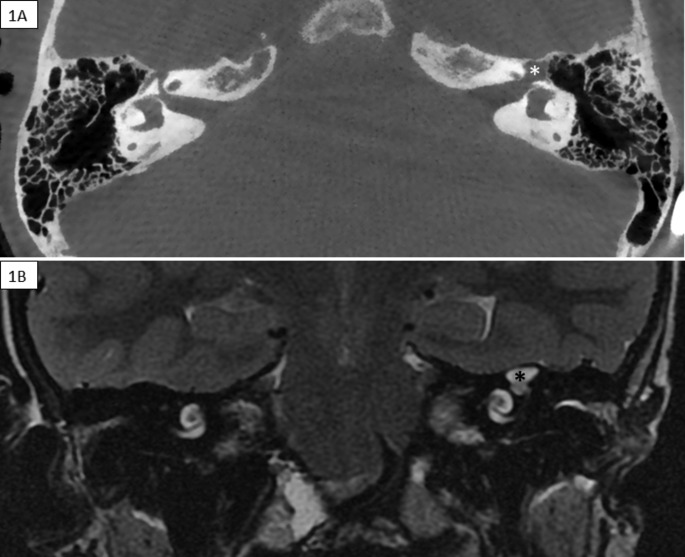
A (Cone Beam CT) and B (MRI, T2 sequence): Fluid ectasia in the left geniculate ganglion (*) in continuity with the tympanic and labyrinthine segments of the facial nerve. There are no bony defects communicating the ipsilateral temporal fossa
